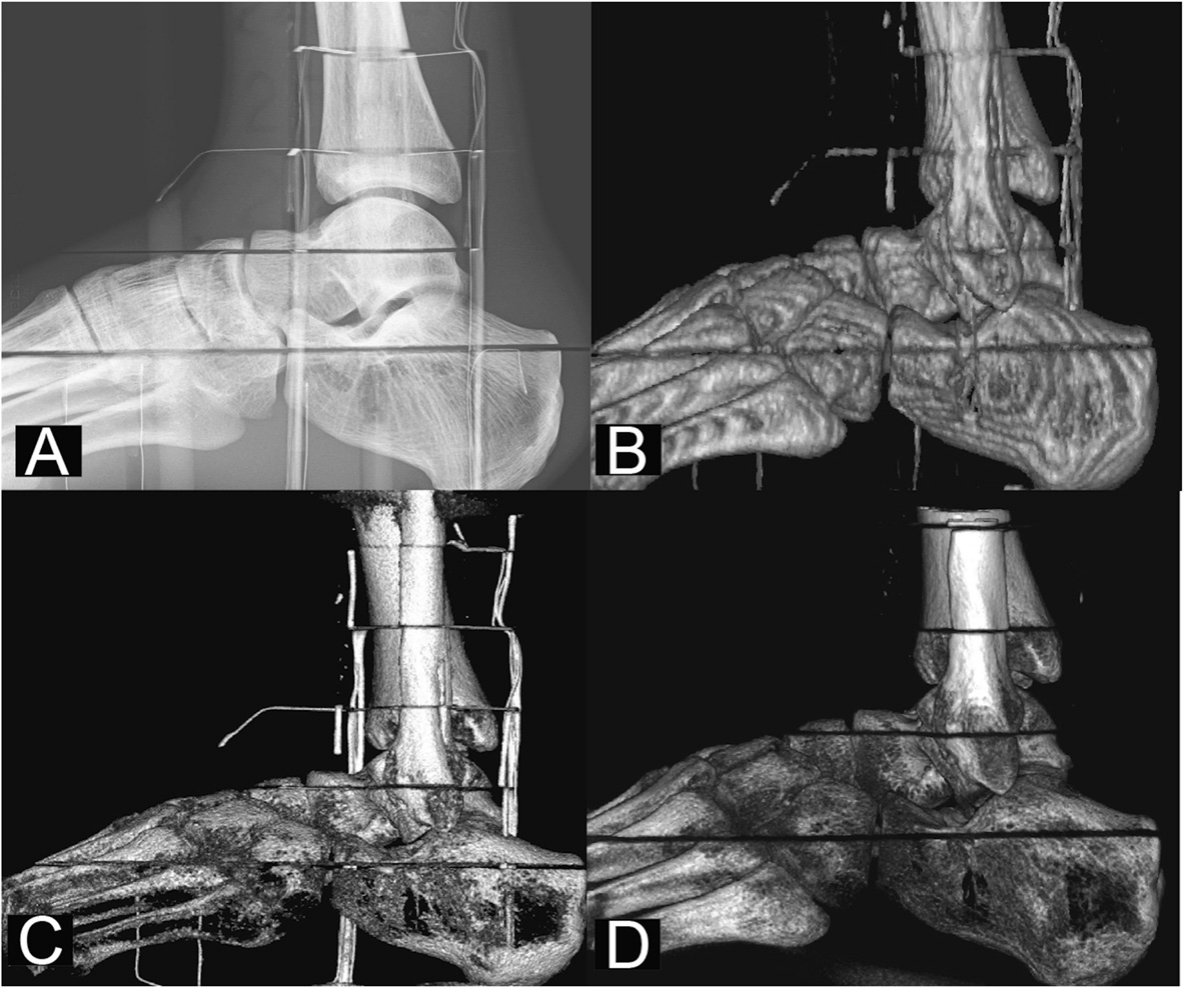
Sagittal ankle projections using the Shimadzu FH21-HR radiography device (A), the Siemens Sensation Open MSCT device (B), the NewTom 5G CBCT device (18 × 16 cm FOV) (C) and the Planmed Verity CBCT device (D).
